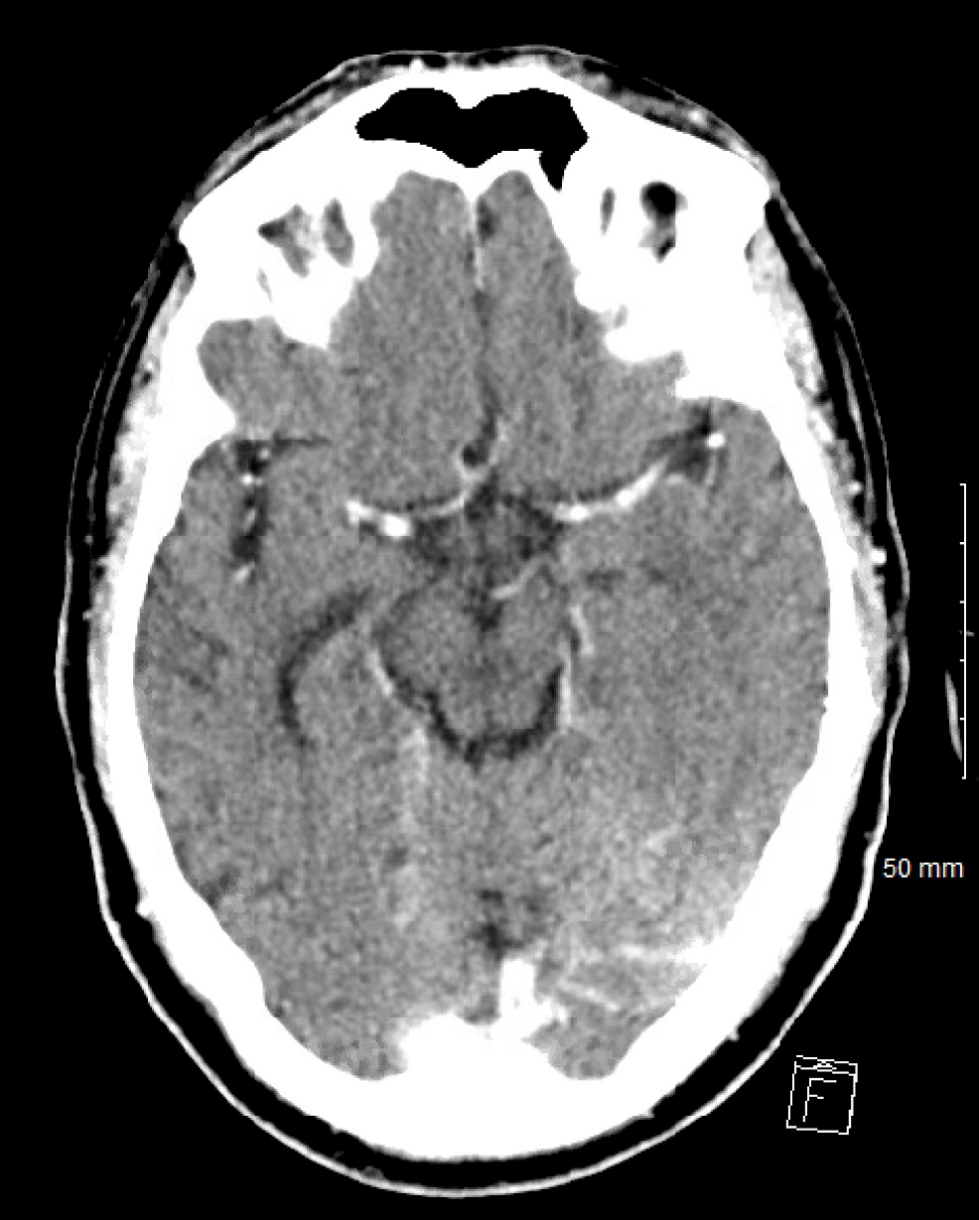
CT head with intravenous contrast showing complete resolution of the intracerebral abscesses following treatment with ceftriaxone. CT: computed tomography
